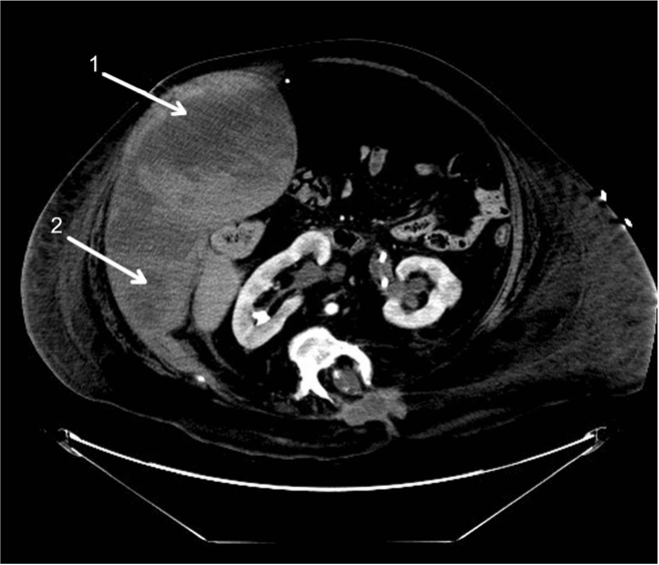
Right rectus sheath hematoma with extension. A CT scan of the abdomen and pelvis revealing a large right rectus sheath hematoma (arrow 1) with extension into the lateral abdominal wall (arrow 2).
